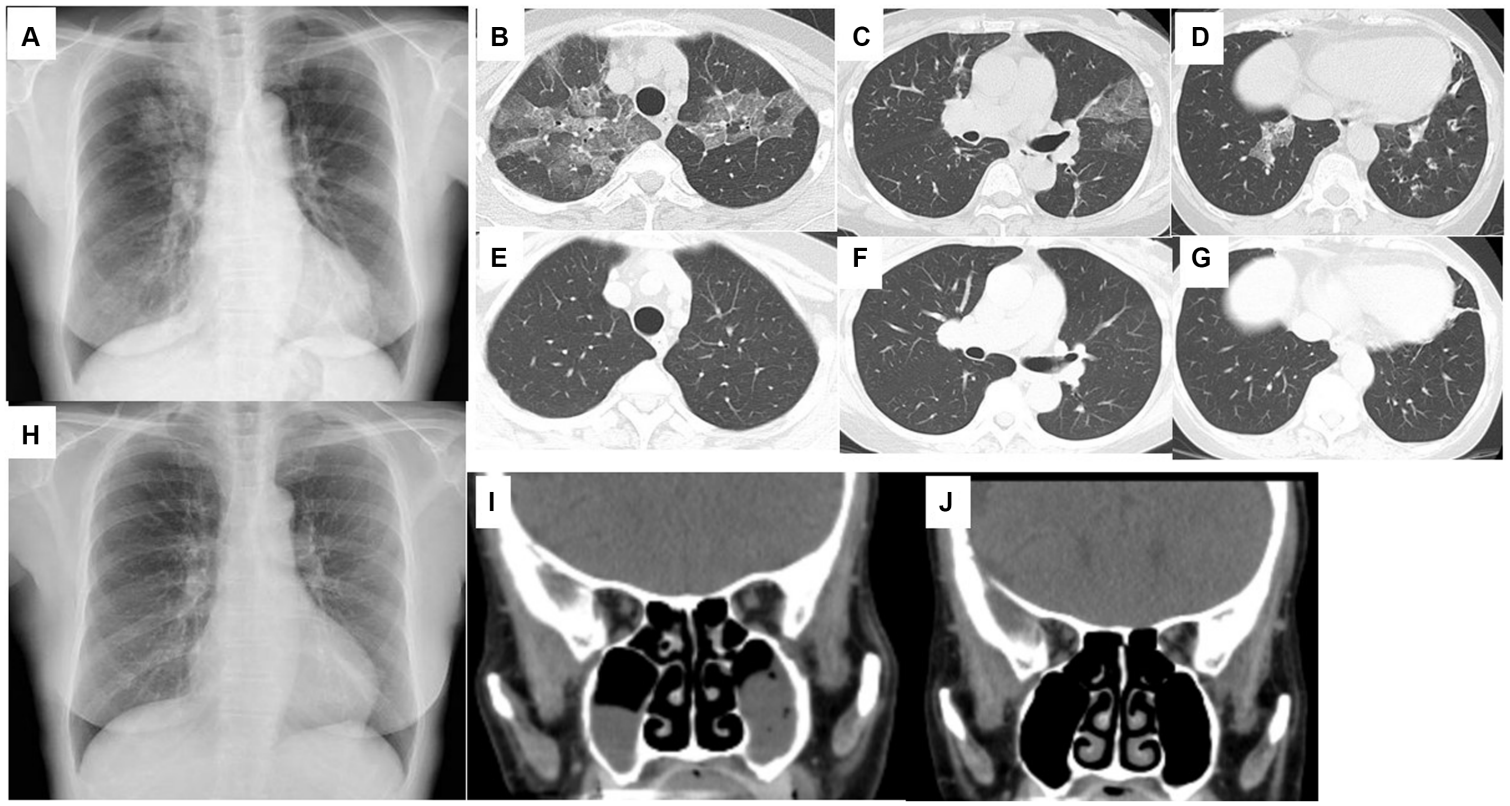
Chest X-ray (A) and chest CT (B–D) on the day of the first visit to the hospital. Bilateral ground-glass opacities in the upper lobes and bronchial wall thickening in the lower lobes. Chest X-ray on day 10 from starting therapy (H). Bilateral ground-glass opacities disappeared. Chest CT performed 1 month after starting therapy (E–G). Bilateral ground-glass opacities and bronchial wall thickening disappeared. Sinus CT on the day of the first visit to the hospital (I). Bilateral sinusitis exists in the maxillary sinus. Sinus CT 3 months after starting therapy (J). Bilateral sinusitis was improved.
